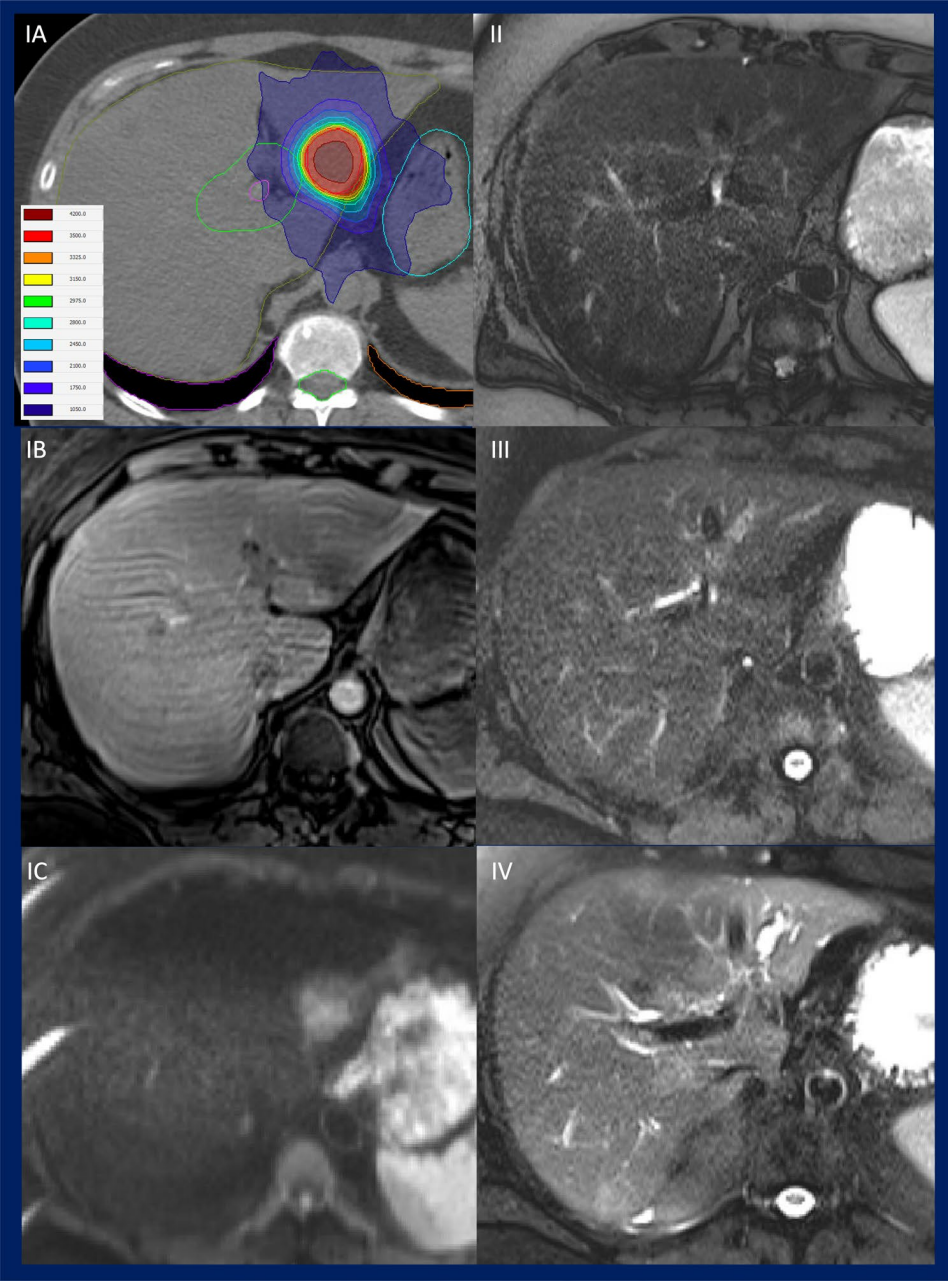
The figure depicts an exemplified longitudinal course of bile duct expansion with the area of the liver shown at approximately the same height: Left column: Treatment planning CT scan for SBRT of a liver metastasis in the left liver lobe with indication of the PTV (red) and the left part of the cHBT (green) within the liver (dark yellow) (IA). Treatment planning MRI scan with a contrast enhanced T1-weighted sequence (IB) and diffusion-weighted MRI (IC). Right column: Longitudinal course of an increase of peritumoural bile duct dilatation, as observed on T2-weighted MRI scans taken at the time of treatment planning (II), at the follow-up three months after SBRT (III) and at the 12-months follow-up after SBRT (IV)
